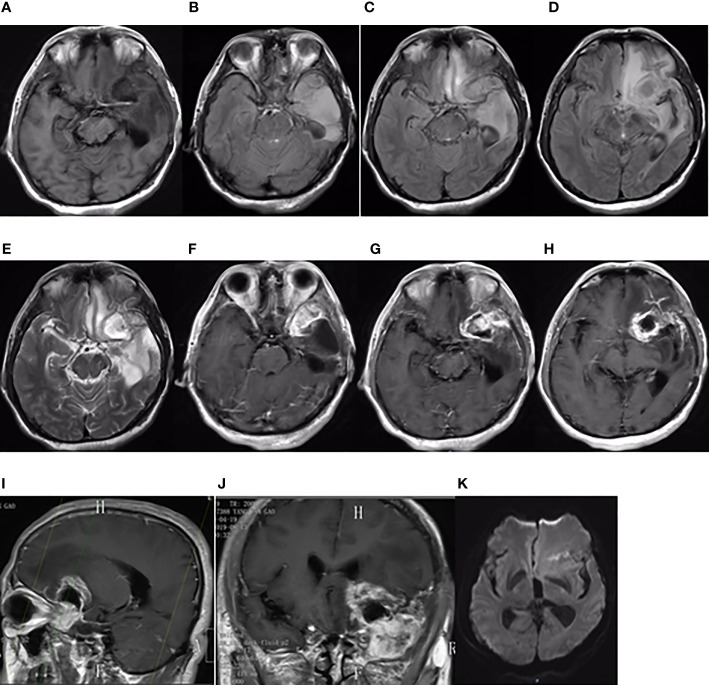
Brain MRI findings on August 13, 2019. Brain MRI showing a new focus in the postoperative residual cavity of the left temporal lobe. (A) The axial plane of T1-weighted MRI. (B–D) The axial planes of T2-weighted FLAIR MRI. (E) The axial plane of T2-weighted MRI. (F–H) The axial planes of enhanced T1-weighted MRI. (I, J) The sagittal and coronal planes of enhanced T1-weighted MRI. (K) The axial plane of diffusion-weighted MRI.
